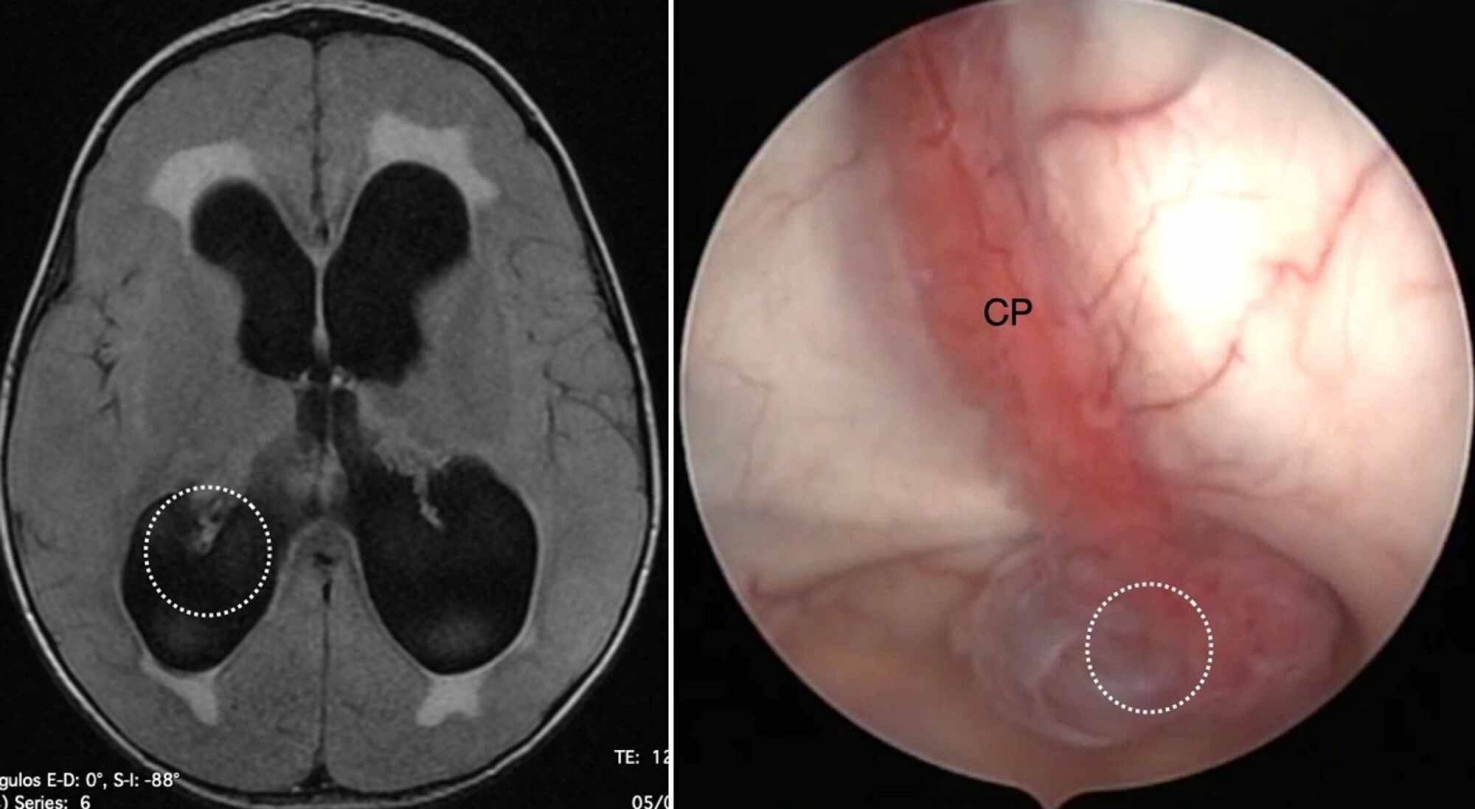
The type IV anatomic variation of the glomus of choroid plexus (GCP). Type IV GCP is classified as immobile and with no elongation. On both sides, the GCP (indicated by the white dotted circle) is close to it fixation near of pulvinar nuclei of the thalamus, as observed in an axial view on T1-weighted magnetic resonance imaging (left). In this endoscopic view of the left lateral ventricle, the GCP is a rounded tuft and unsuitable for resection by our technique. CP, choroid plexus.
